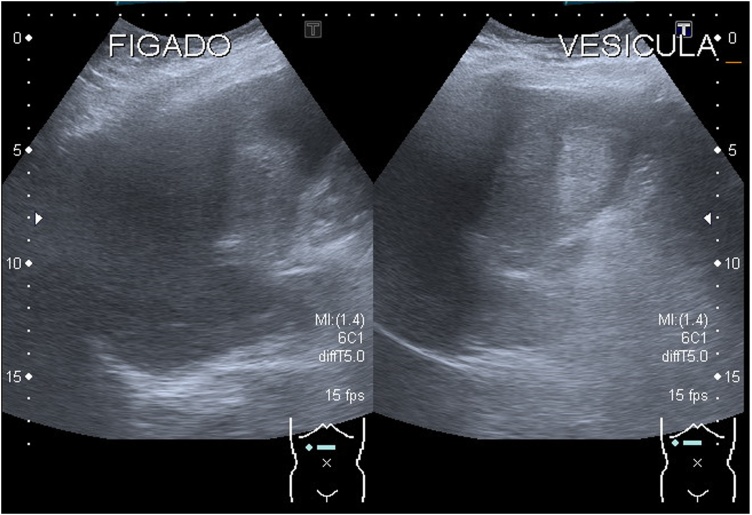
Abdominal Ultrasound image showing a heterogenic content of the gallbladder, with no acoustic shadow. “FIGADO” means liver. “VESICULA” means gallbladder.
